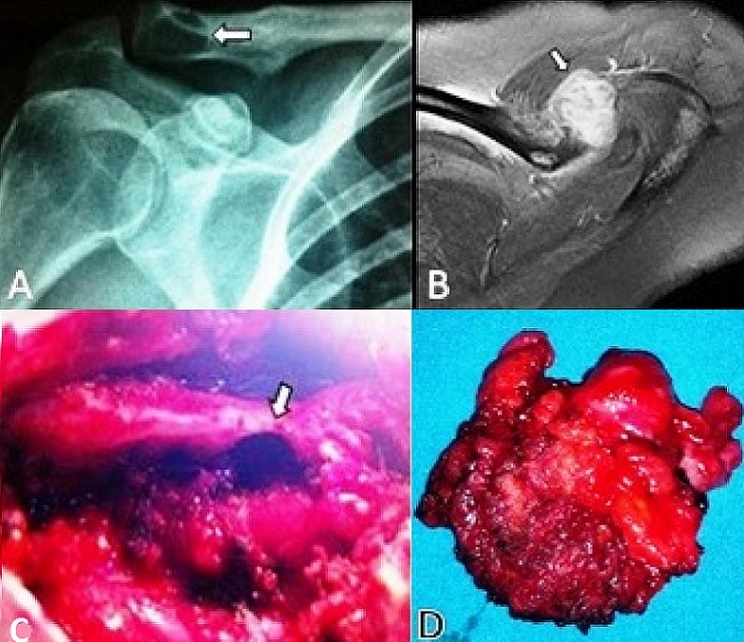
(A) plain radiograph of left shoulder demonstrates a radiolucent area at the lateral edge of the clavicle(arrow); (B) axial T1-weighted MR image with fat suppression after intravenous gadolinium administration shows an enhancing mass with cortical disruption and invasion into the medullary space (arrow); (C) the intra-op image shows severe osseous destruction of the clavicle (arrow); (D) macroscopic view of the excised desmoid tumor
